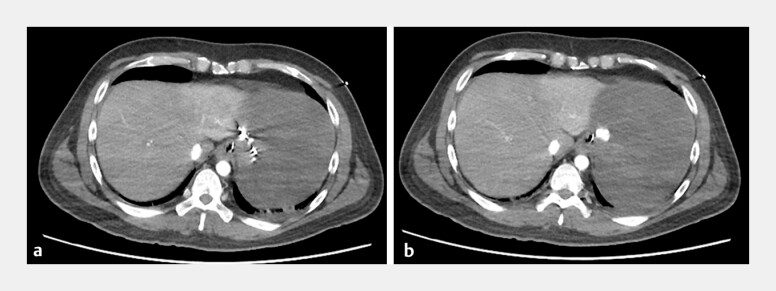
Dynamic abdominal computed tomography revealed a left gastric artery pseudoaneurysm at the esophagogastric junction.
